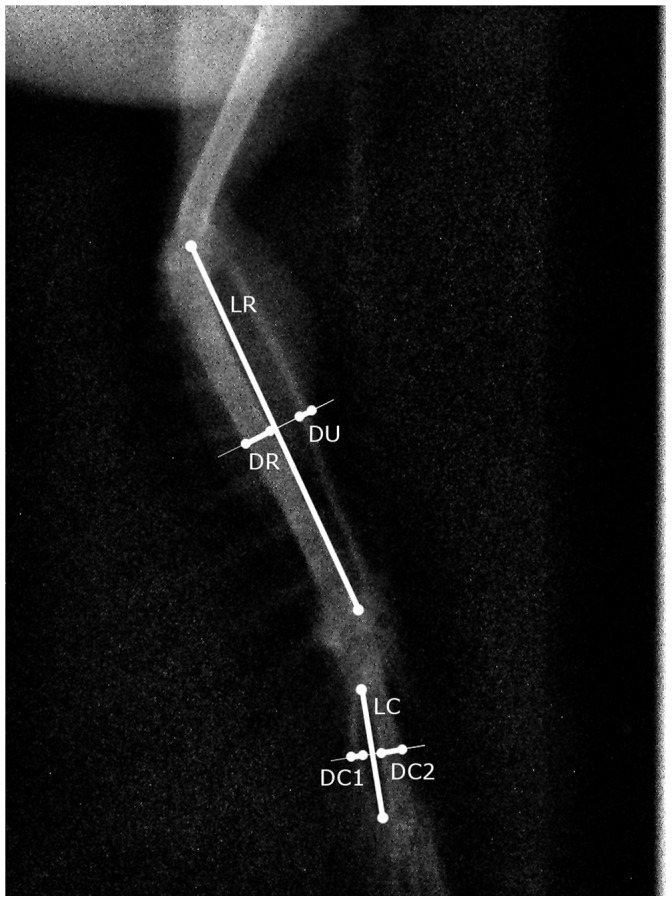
X-ray image of the skeletal structure of a wing with the position of all 12 landmarks and associated wing traits indicated. LR, length radius; DR, diameter radius; DU, diameter ulna; LC, length of the carpometacarpal window; DC1 and DC2, diameter of both bony structures of carpometacarpus.
